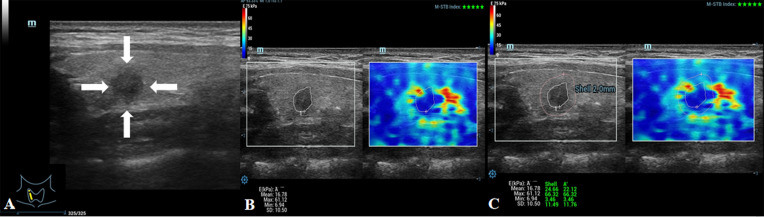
Images showing an ACR-TIRADS 4 TN in a 48-year-old female patient. The pathological diagnosis after surgery was papillary thyroid carcinoma. (A) Conventional ultrasound image of the TN; the arrows point to the TN. (B)
